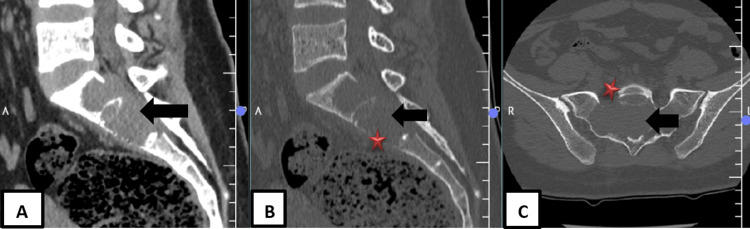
(A) Sagittal soft-tissue CT, (B) sagittal bone-window CT, and (C) axial bone-window CT at sacral level shows a well-defined expansile osteolytic lesion centered in S1–S2 (black arrow) with marked endosteal scalloping, cortical thinning, and mild anterior cortical breach at S2 (red star). The lesion has a narrow zone of transition without periosteal reaction and extends posteriorly into the spinal canal, causing mass effect on the thecal sac.
